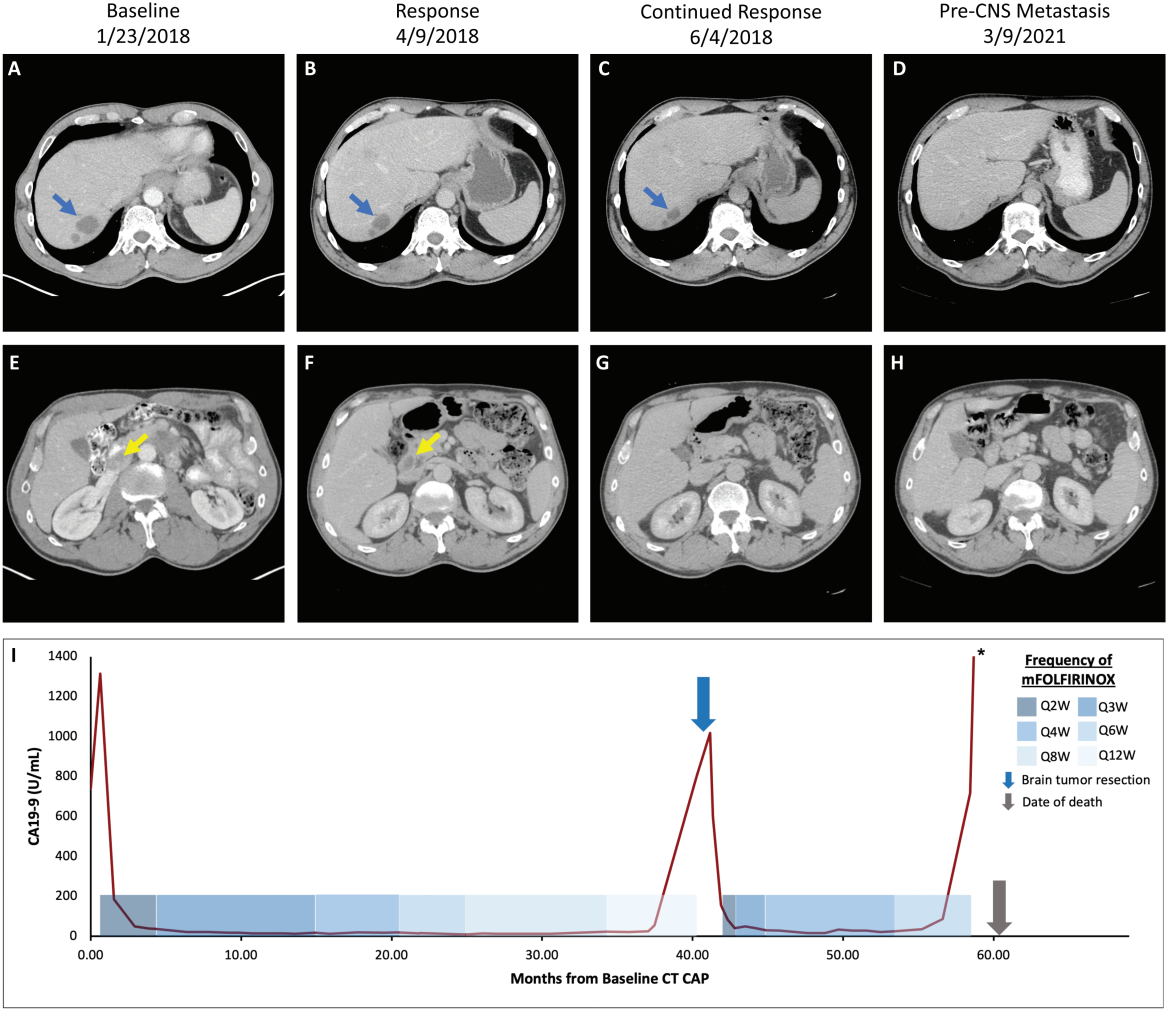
Radiological and biochemical response. Serial contrast-enhanced abdominal CT scans showing the patient’s baseline disease prior to initiation of mFOLFIRINOX (A, E), response to mFOLFIRINOX (B, F), continued response to mFOLFIRINOX (C, G), and sustained response 3 months prior to identification and resection of brain metastasis (D, H). Graphical depiction of CA19-9 levels over time (I) with response to mFOLFIRINOX. Yellow arrow: Primary pancreatic adenocarcinoma. Blue arrow: Metastatic pancreatic adenocarcinoma to hepatic segment VII. Abbreviations: CNS, central nervous system; mFOLFIRINOX, modified fluorouracil, leucovorin, irinotecan, oxaliplatin; CT CAP, computed tomography scan of chest, abdomen, and pelvis; Q, every; W, weeks. *CA19-9 value of 4762 U/mL.
